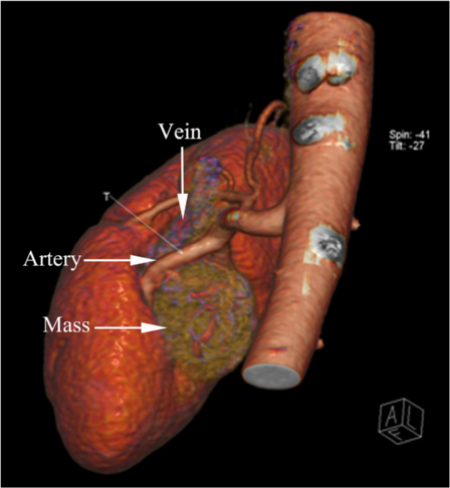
The preoperative computed tomographic angiography (CTA) image of the ectopic adrenal tumor
